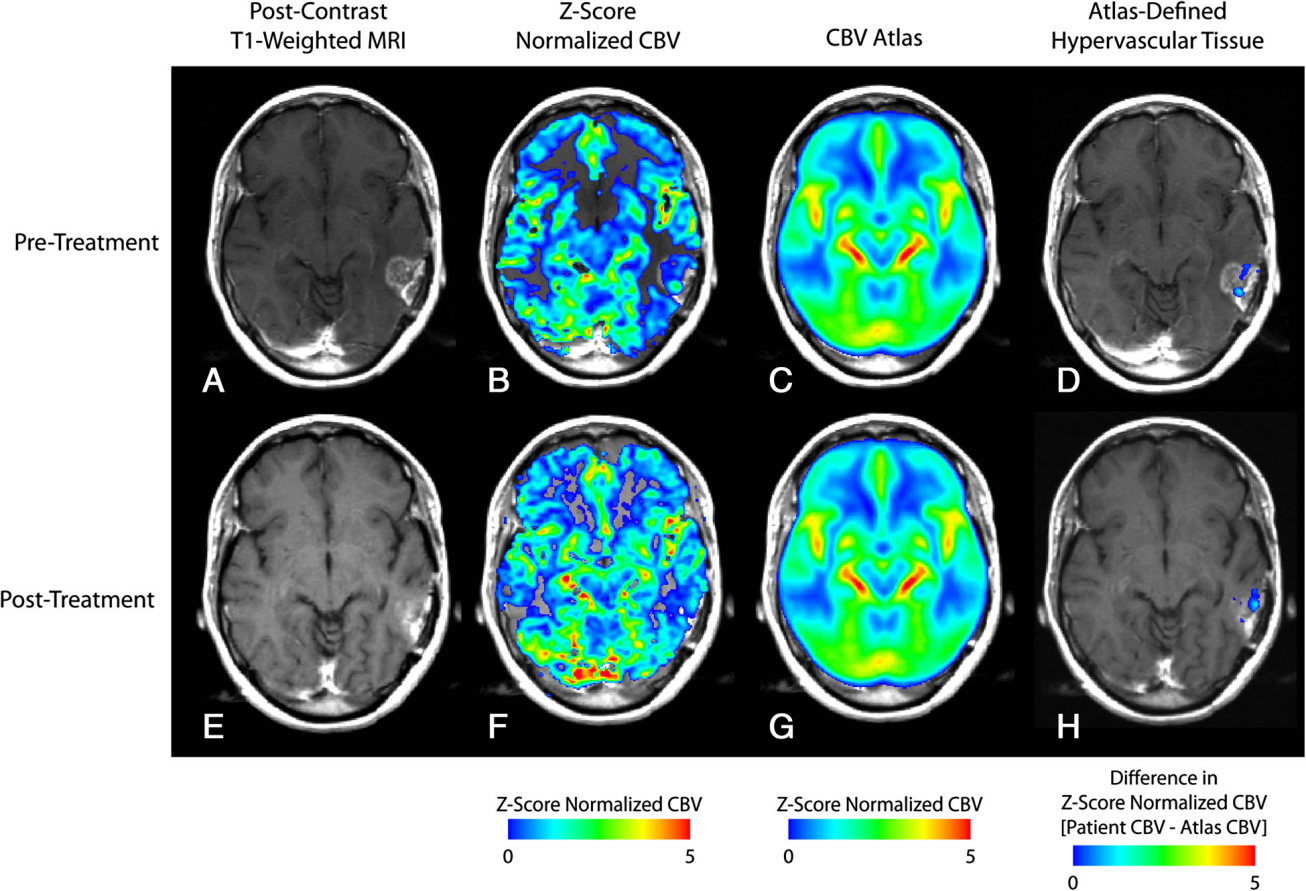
Responder to bevaciuzumab (PFS =114 days; OS =486 days). A) Pre-treatment, post-contrast T1-weighted image. B) Pre-treatment, Z-score normalized CBV. C) CBV atlas. D) Pre-treatment, atlas-defined hypervascular tumor tissue showing a relatively small extent of abnormal vasculature prior to therapy. E) Post-treatment, post-contrast T1-weighted image. F) Z-score normalized CBV. G) CBV atlas. H) Atlas-defined hypervascular tumor tissue showing a small residual volume of abnormal vasculature after administration of bevacizumab.
